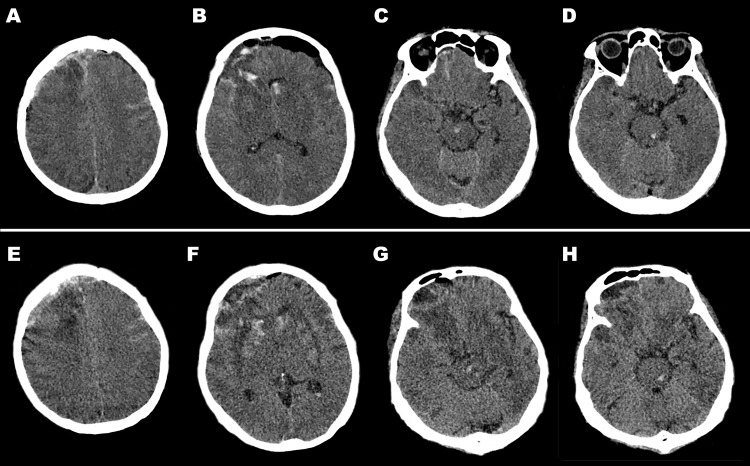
Postoperative CT scan (A-D) Axial CT scan showed subarachnoid hemorrhages in the bilateral frontoparietal sulci and subdural hemorrhage in the right frontal convexity (A), hemorrhage in the surgical bed in the right frontal lobe, and acute hemorrhages in the left caudate head (B), midbrain (C), and left hemipons (D). There was also diffuse cerebral edema, with effacement of the bilateral frontal horns of the lateral ventricles and right cerebral cortical sulci. (E-H) A follow-up CT scan on the first postoperative day showed progression of cerebral edema, with the brain parenchyma now occupying the previous postoperative cavity in the right anterior cranial fossa and the frontal horns of both lateral ventricles (more in the right) still compressed. An increase of the subdural hemorrhage in the right frontal convexity is noted, with no change in the subarachnoid hemorrhages in the bilateral frontoparietal sulci (E). There were hemorrhages in the bilateral caudate and lentiform nuclei (F), with no significant change in the amount of hemorrhages in the midbrain (G) and left hemipons (H). CT: computed tomography
